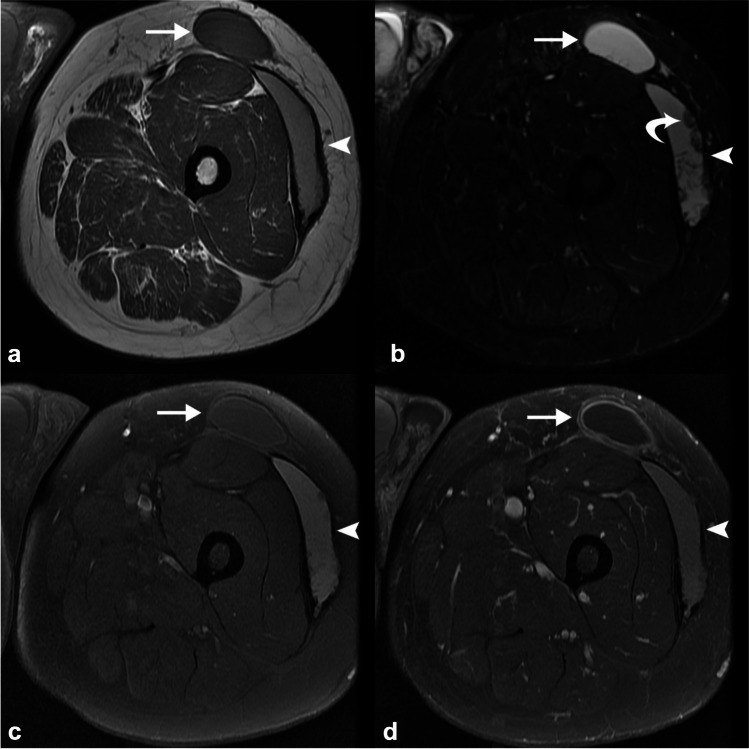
Morel-Lavallée lesions mimicking abscess and hematoma. Fifty-five-year-old man with left thigh masses and worsening pain. Axial T1 (a), STIR (b), and T1 FS pre- (c) and post-contrast (d) MRI images demonstrate two perifascial left thigh subcutaneous fluid collections. The anterior collection (*arrow*) is T1 isointense, STIR hyperintense, and has rim enhancement, which can mimic the appearance of an abscess. The lateral collection (*arrowhead*) is predominantly T1 and STIR hyperintense with a hypointense rim and areas of mural nodularity (retracted clots, *curved arrow*, c), which could mimic a hematoma. However, these represent type I (anterior) and type III (lateral) Morel-Lavallée lesions with a history of subacute trauma subsequently elicited
